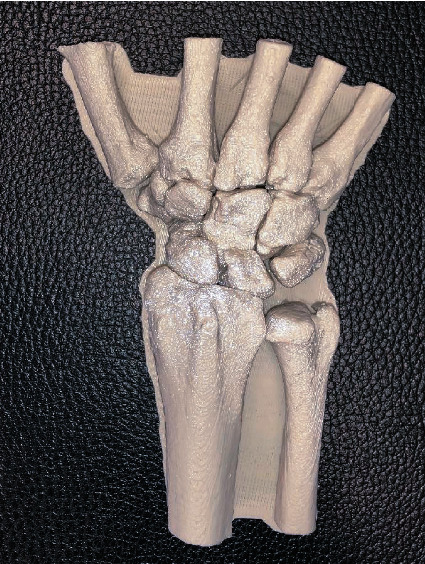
1 : 1 model based on 3D-Computertomography (CT) data of the carpal bones printed on an FDM-printer. This model allows analysis of intracarpal relationships und surgical training.
